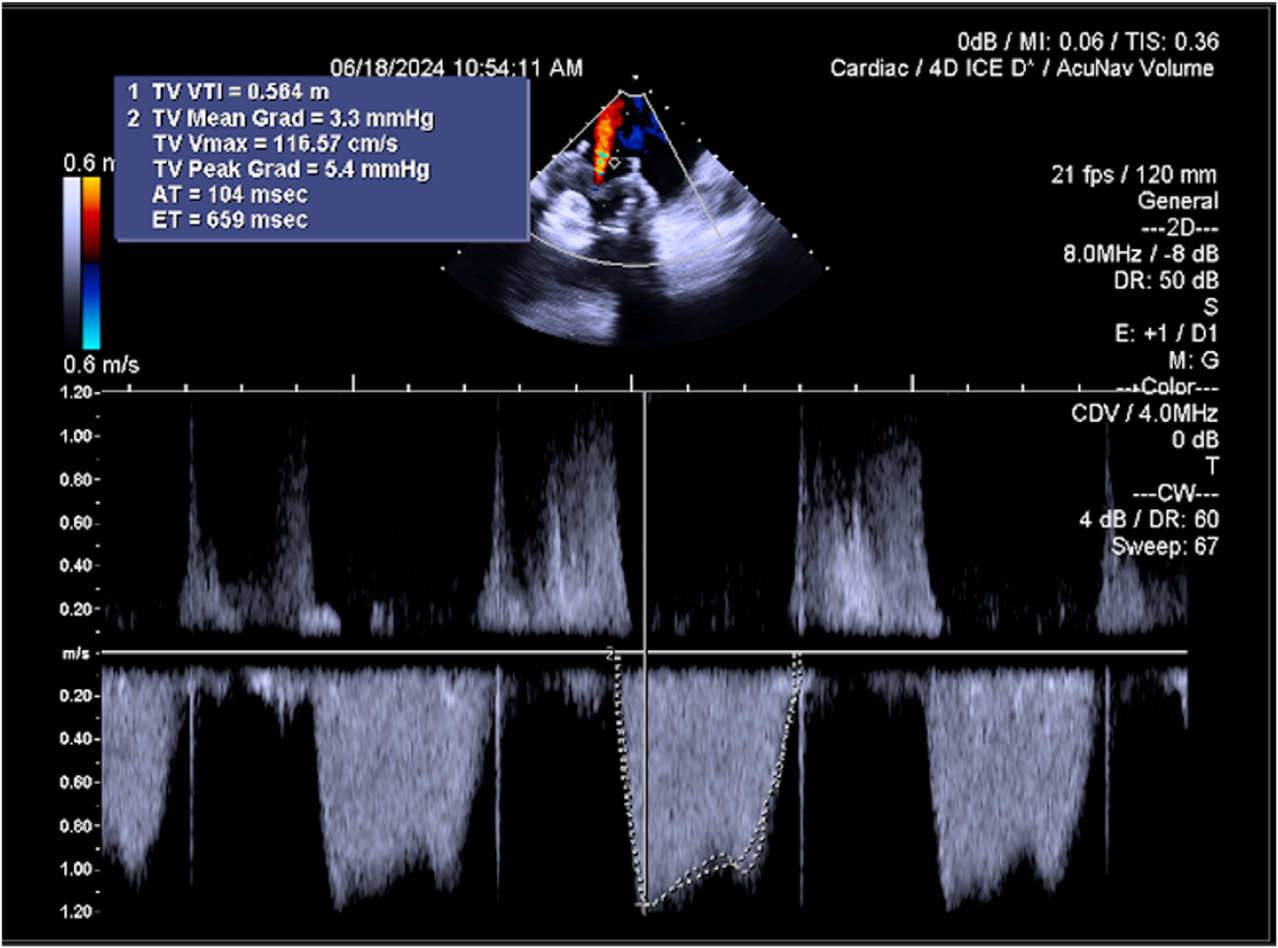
Final intracardiac echocardiographic imaging after PVL closure. Abbreviations: AT, acceleration time; CDV, Color Doppler Velocity; CW, continuous wave; ET, ejection time; PVL, paravalvular leak; TV, tricuspid valve; Vmax, peak aortic valve velocity; VTI, velocity time interval; 2D, two-dimensional.
